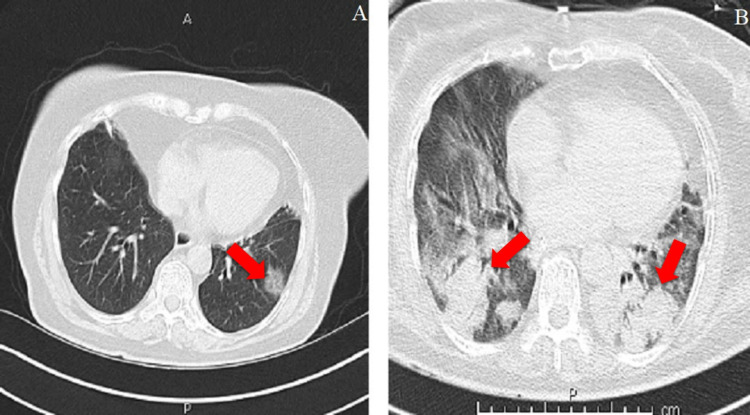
Chest CT scan of a 66-year-old female patient. A. Third chest CT image taken 14 days after the first and seven days after the second CT showing increased GGO in the upper lobes. B. Air bronchograms and GGO accompanying consolidations in the lower lobes. GGO: ground-glass opacities.
